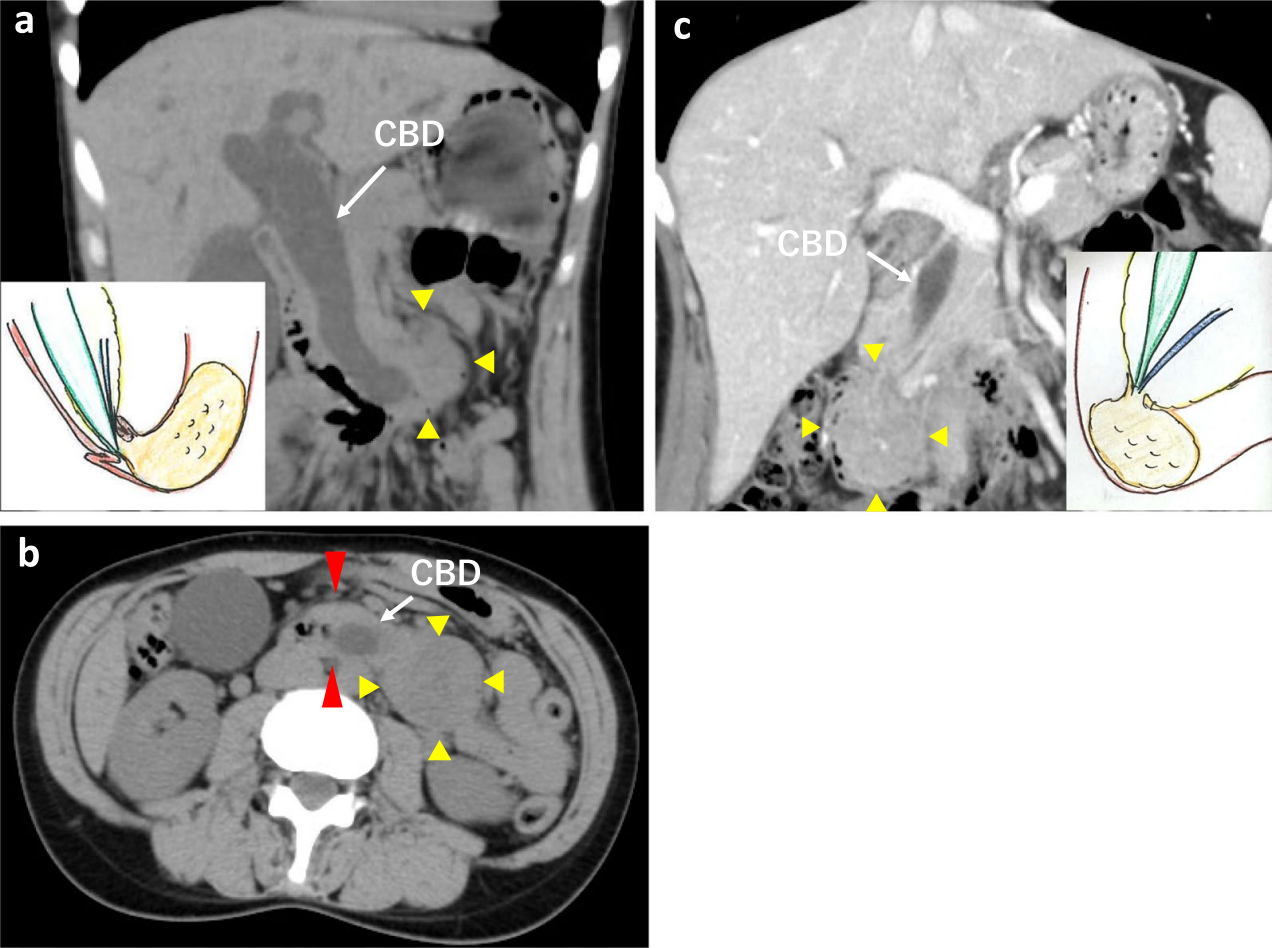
Contrast-enhanced CT. a, b A 60-mm hypovascular tumor (yellow arrowhead) in the third part of the duodenum and a left lateral shift of the dilated common bile duct (CBD). The obstruction was caused by duodenal wall ampulla-side partial intussusception (red arrowhead). c A 60-mm hypovascular tumor (yellow arrowhead) that shifted from the third part of the duodenum to the second part as well as improvement in the dilated common bile duct (CBD)
